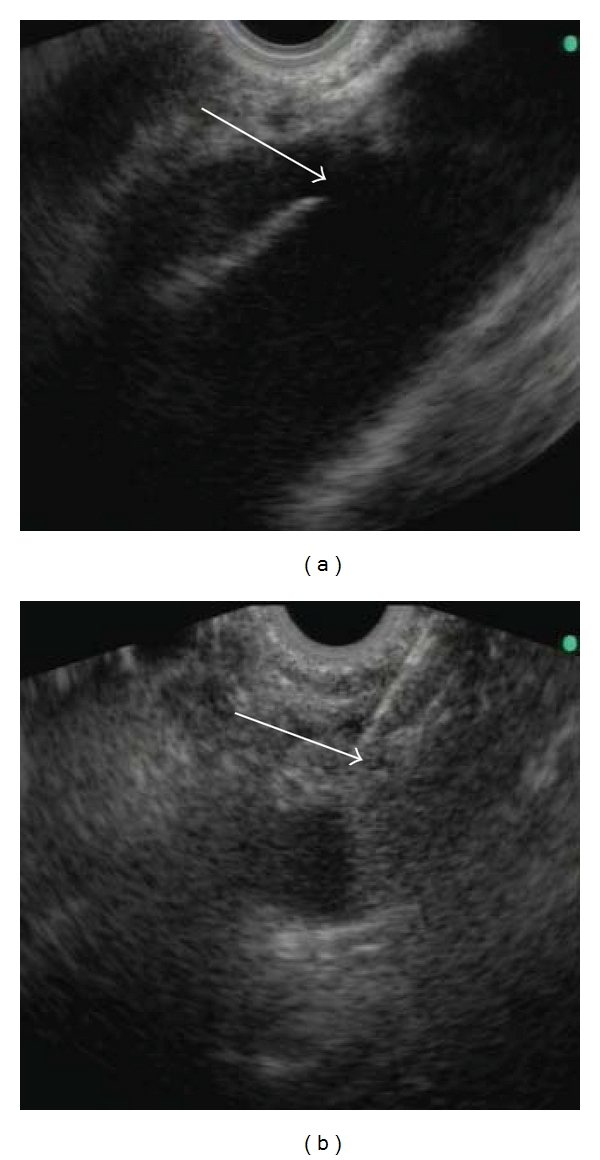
Endoscopic ultrasound-guided celiac plexus neurolysis: (a) typical location of the plexus with the celiac trunk (arrow) at the aorta, (b) puncture of the celiac plexus with administration of alcohol under endoscopic ultrasound guidance.
